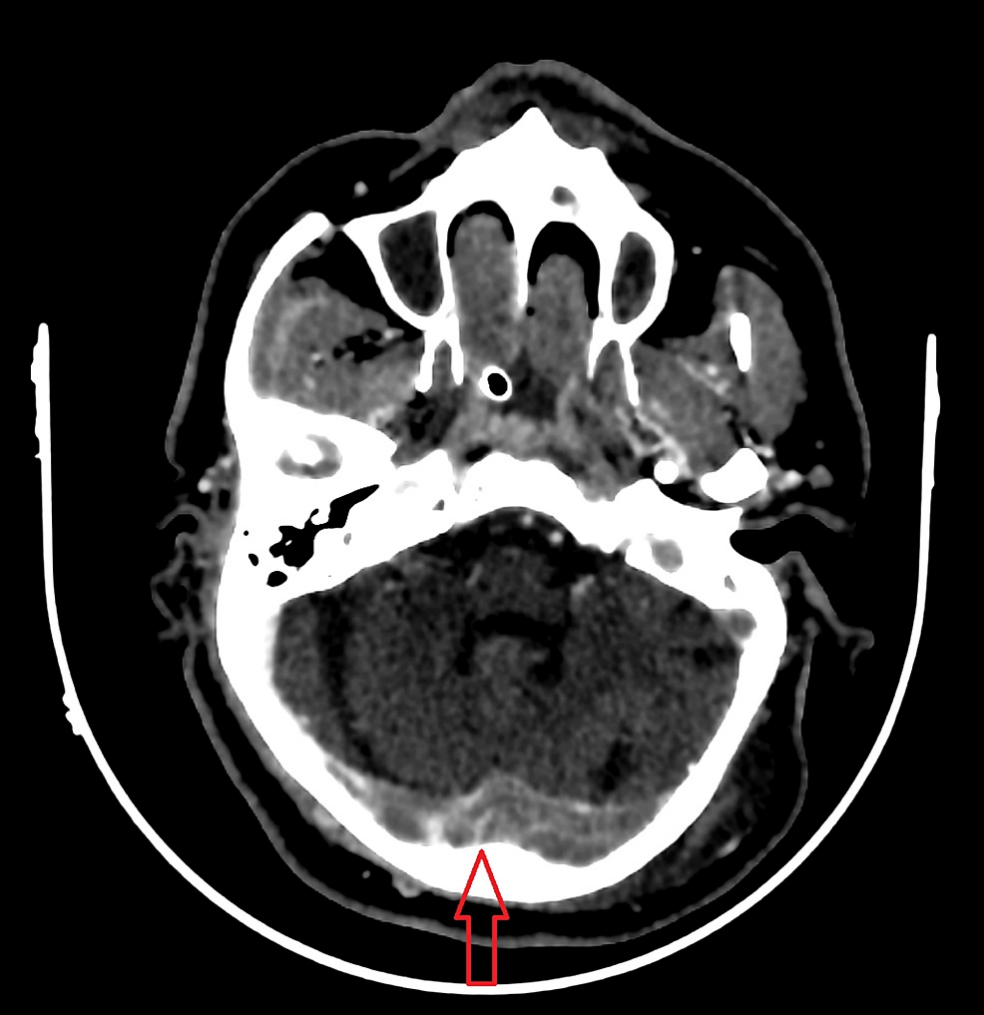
A CT-venogram of the head showing extensive acute dural sinus thrombosis (red arrow).
